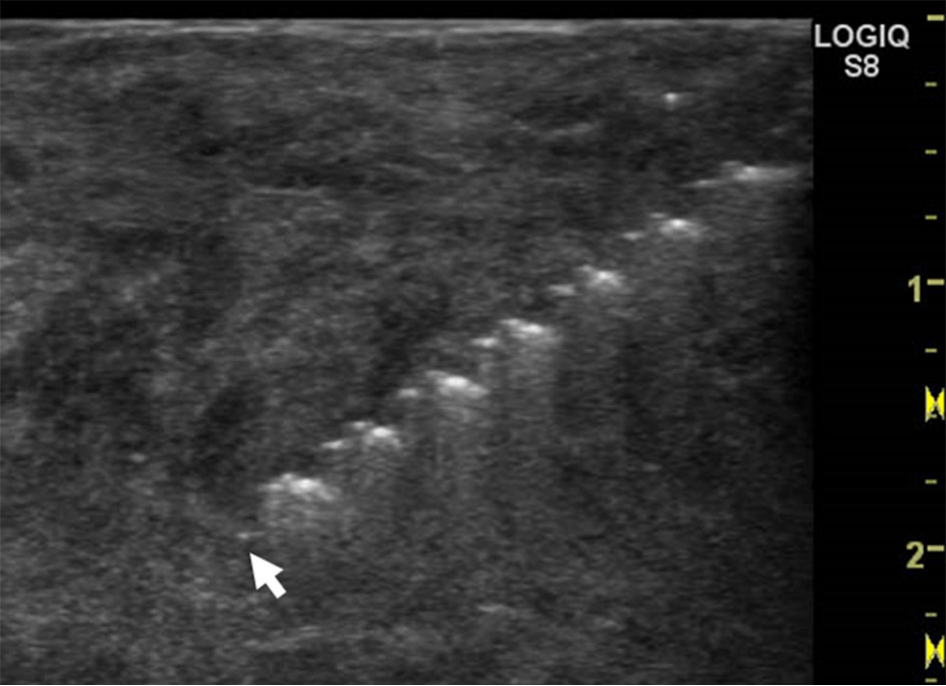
During this pass of DNAB, the drill tip is visualized using ultrasonography. The tip (*arrowhead*) and helical ditch of the drill are clearly observed
